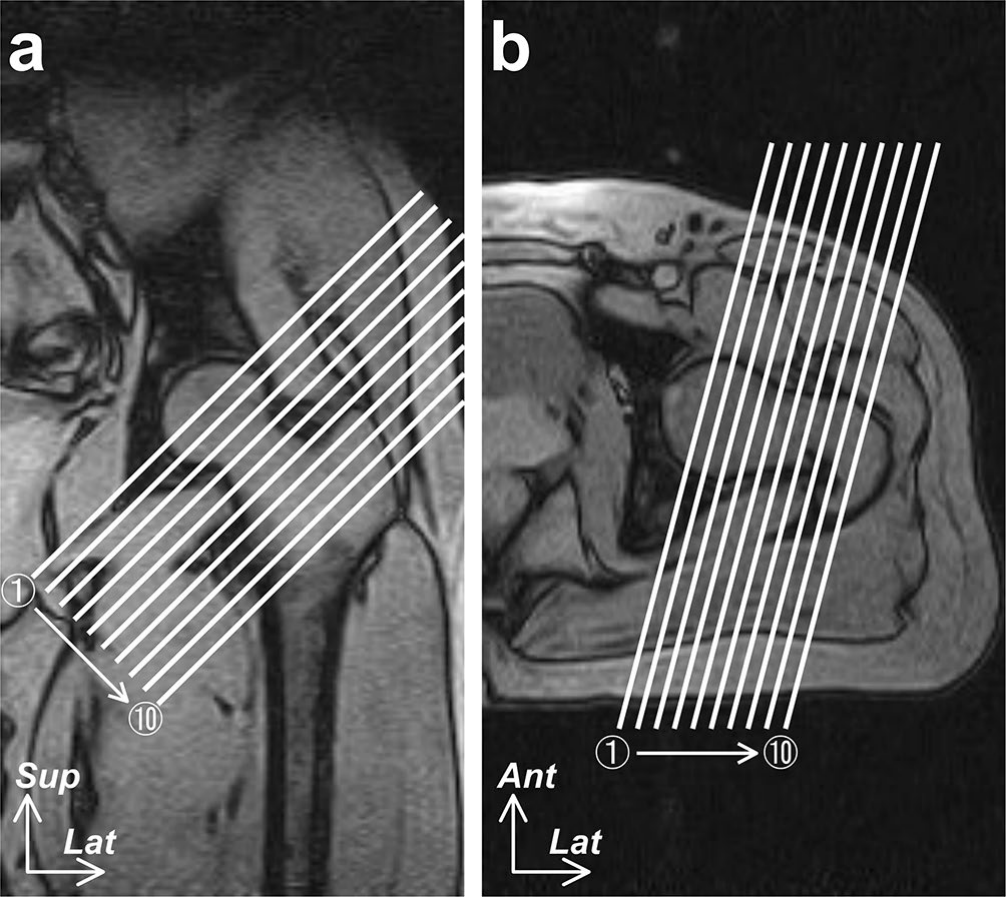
Slice location and imaging direction of the magnetic resonance imaging. Coronal (a) and horizontal (b) sections of the left hip in extension. The locations and directions of the slices are indicated by the white lines. *Ant* = anterior, *Lat* = lateral, and *Sup* = superior.
